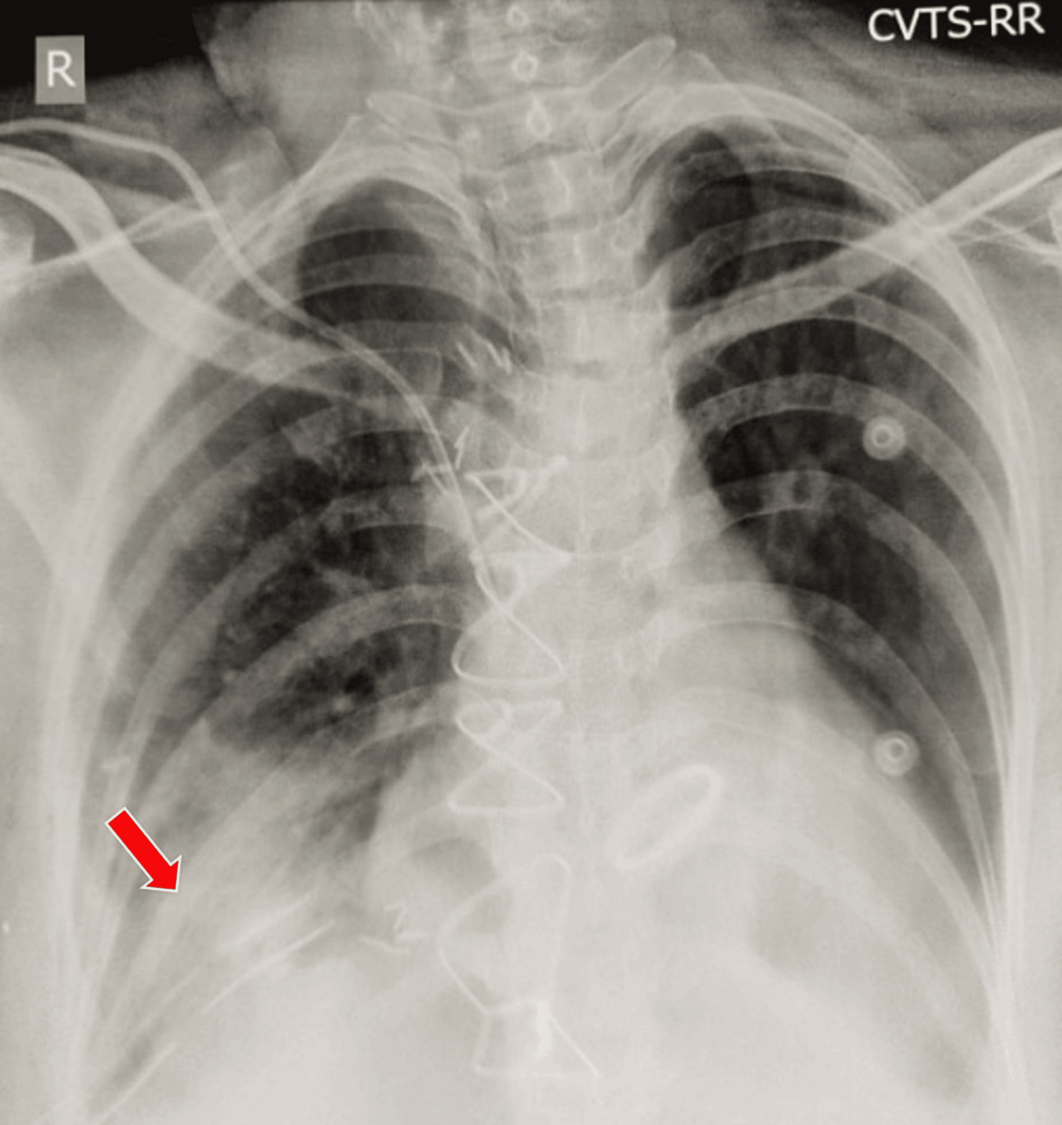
Chest radiograph immediately post-diaphragmatic plication showing no significant lesion resolution (red arrow).
